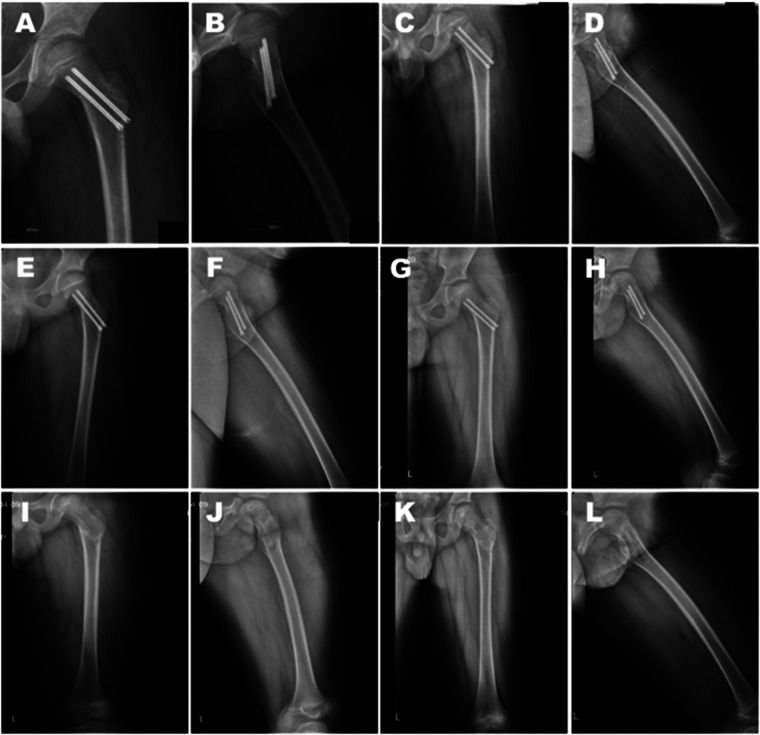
Postoperative anteroposterior and lateral x-ray radiographs. (A–H) The patient was examined at 1 day (A,B), 1.5 months (C,D), 4 months (E,F), and 10 months (G,H) after surgery, as well as 1 day (I,J) and 1 month (K,L) after implant removal.
